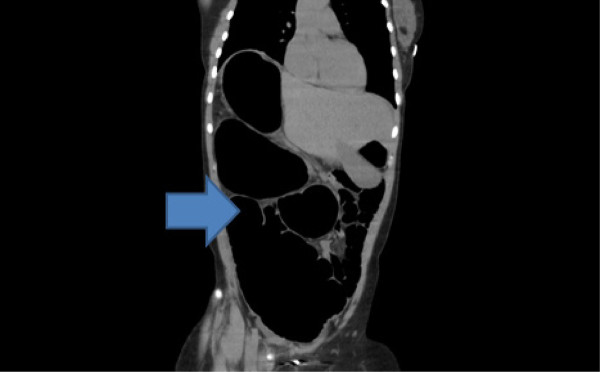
Computed tomography scan of the abdomen showing the distal colon collapsed with a transition point in the mid abdomen suggestive of a volvulus or band.
